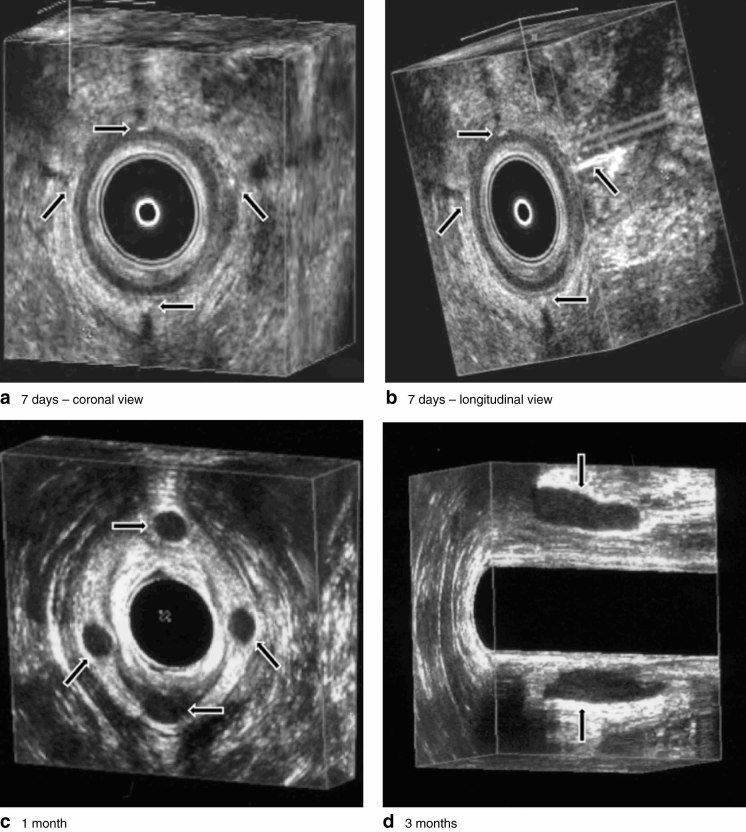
Endoanal ultrasound imaging at a,b 7 days (a coronal view, b longitudinal view), c 1 month and d 3 months after implantation of Gatekeeper™ prostheses (arrows)
